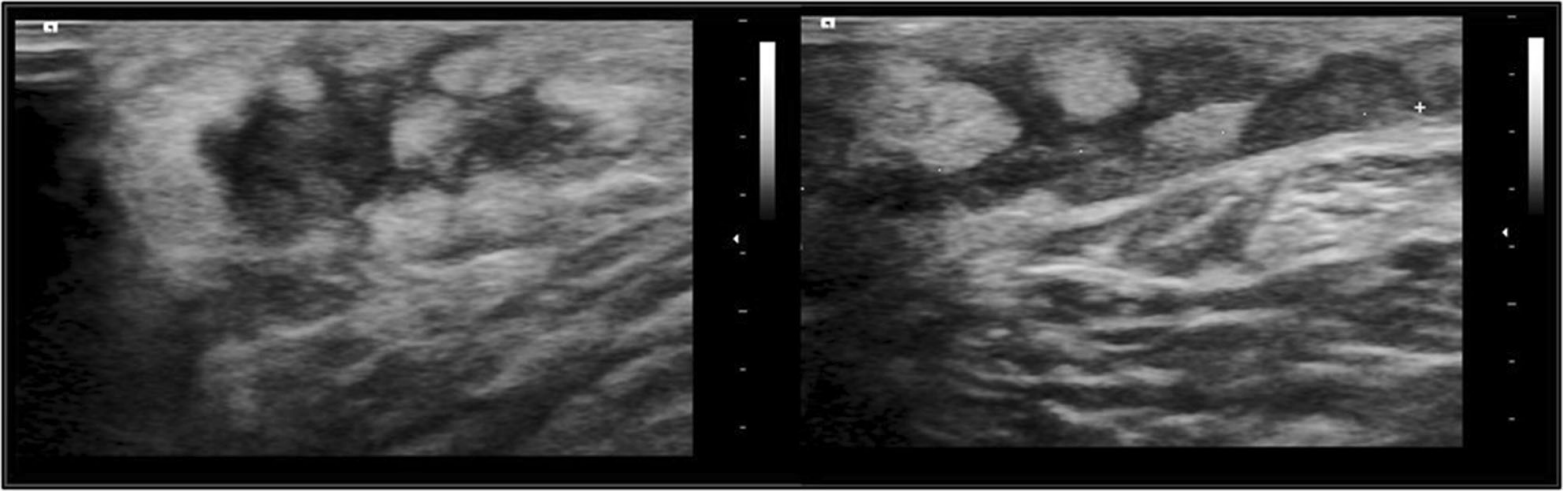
Several subcutaneous oedemic lesions were found in an ultrasound examination autumn 2022. The etiology of the lesions was unsure. Although the cause of these lesions was uncertain, no biopsy was taken at the time. However, a few weeks later, a positron emission tomography scan showed multiple ^18^F-Fluorodeoxyglucose activities in the same areas
